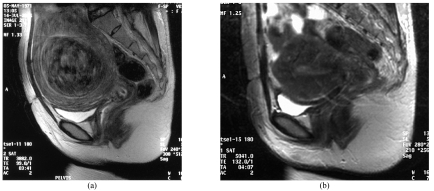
A 45-year-old woman presented with a two-month history of menorrhagia which required repeated blood transfusion. She was advised to have surgery but declined. Her initial MRI (a) showed the presence of a large submucosal fibroid. Due to her clinical status, a decision was taken to do a UAE. Her follow-up MRI at 1 year (b) shows complete absence of the fibroid.
